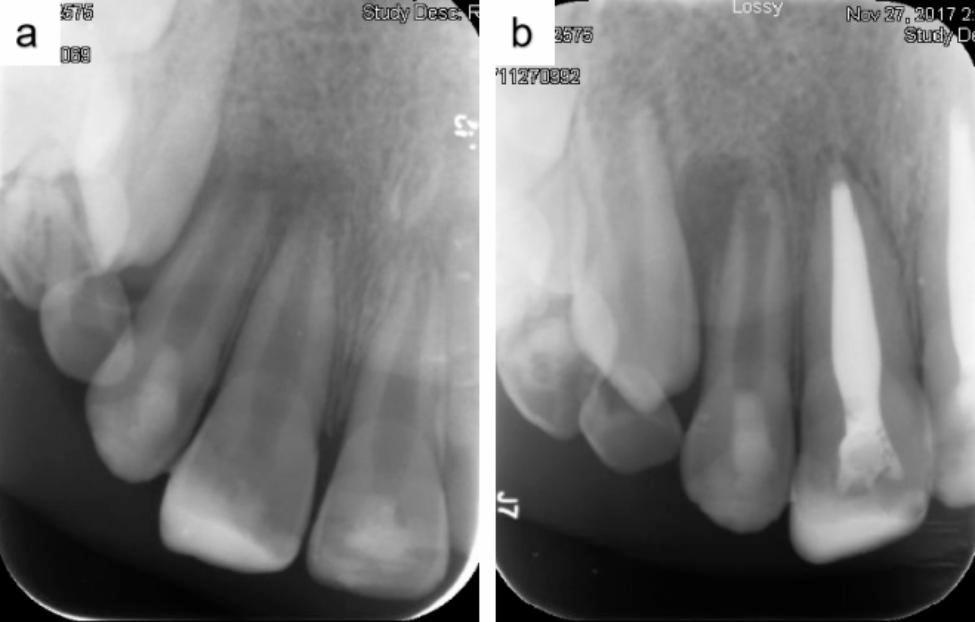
Periapical radiographs over the 9-month follow-up period. (a) Postoperative periapical radiograph of tooth 12 showing an incompletely developed root with a periapical lesion. (b) Postoperative periapical radiograph at the 15-month follow-up showing sustained periapical lesion even though a complete root development is achieved
